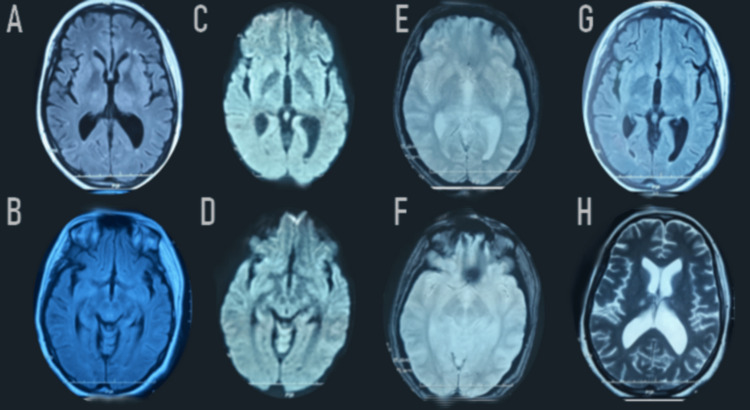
Remarkable regression of the FLAIR-weighted (A-B-G) and diffusion-weighted (C-D) bilateral hypersignal in the thalamus. Extinction of the bilateral signal abnormalities in the external capsule, posterior limb of the internal capsule, and in the pons. Axial T2*-weighted images (E-F) showed bilateral thalamic hemorrhagic lesions and in the posterior part of the putamen, as well as the presence of minimal intraventricular subarachnoid hemorrhage in the falx cerebri and cerebellar tentorium. FLAIR: Fluid-Attenuated Inversion Recovery; T2: T2-weighted imaging; DWI: Diffusion Weighted Imaging.
